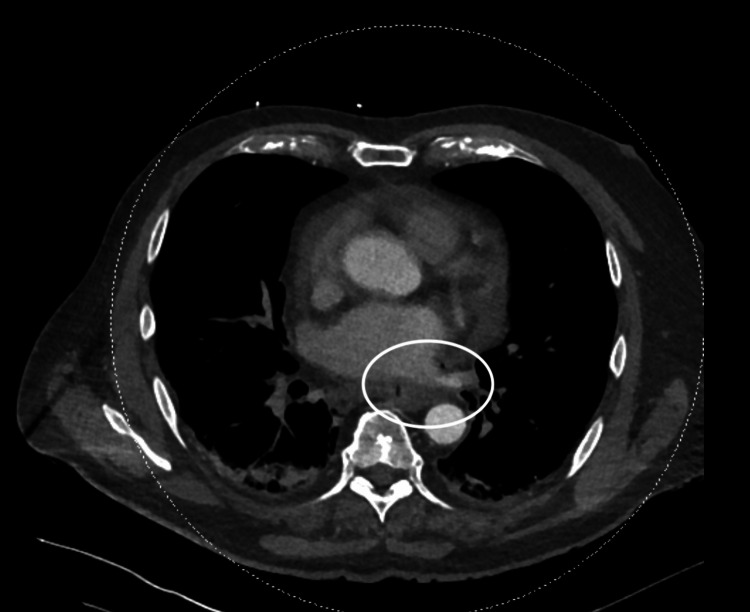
Computed tomography angiography showing pneumomediastinum
